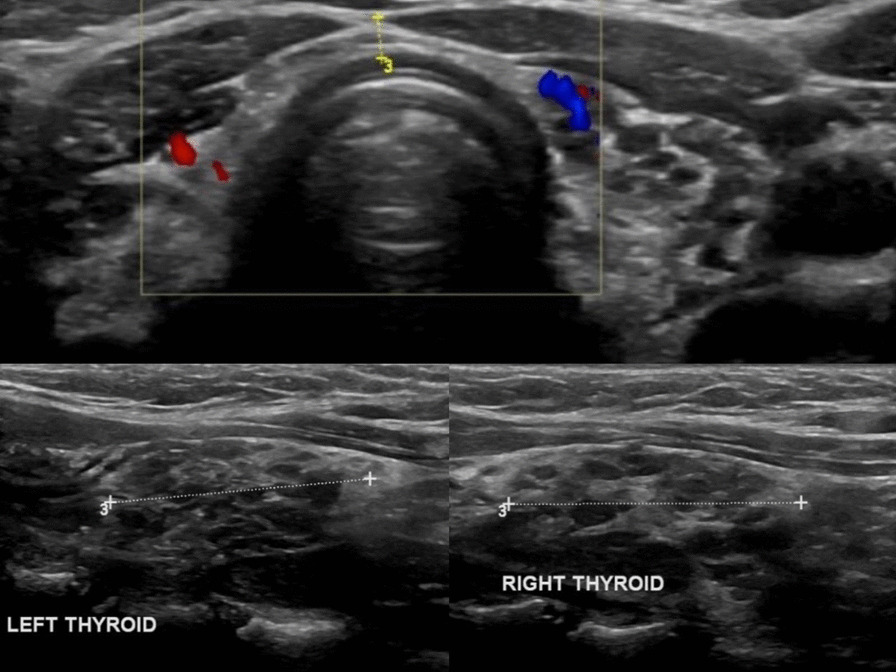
Ultrasound of the thyroid showing diffuse cystic changes replacing much of the normal thyroid tissue with a “Swiss cheese” appearance
